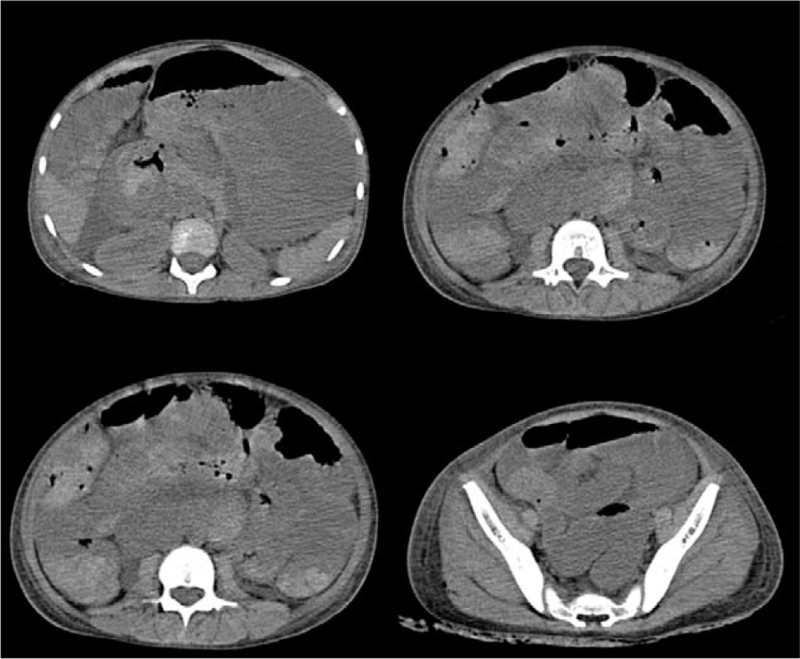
Abdominal CT scanning revealed changes in the stomach, arc-shaped fluid and gas shadows, and peritoneal effusion. CT = computed tomography.
